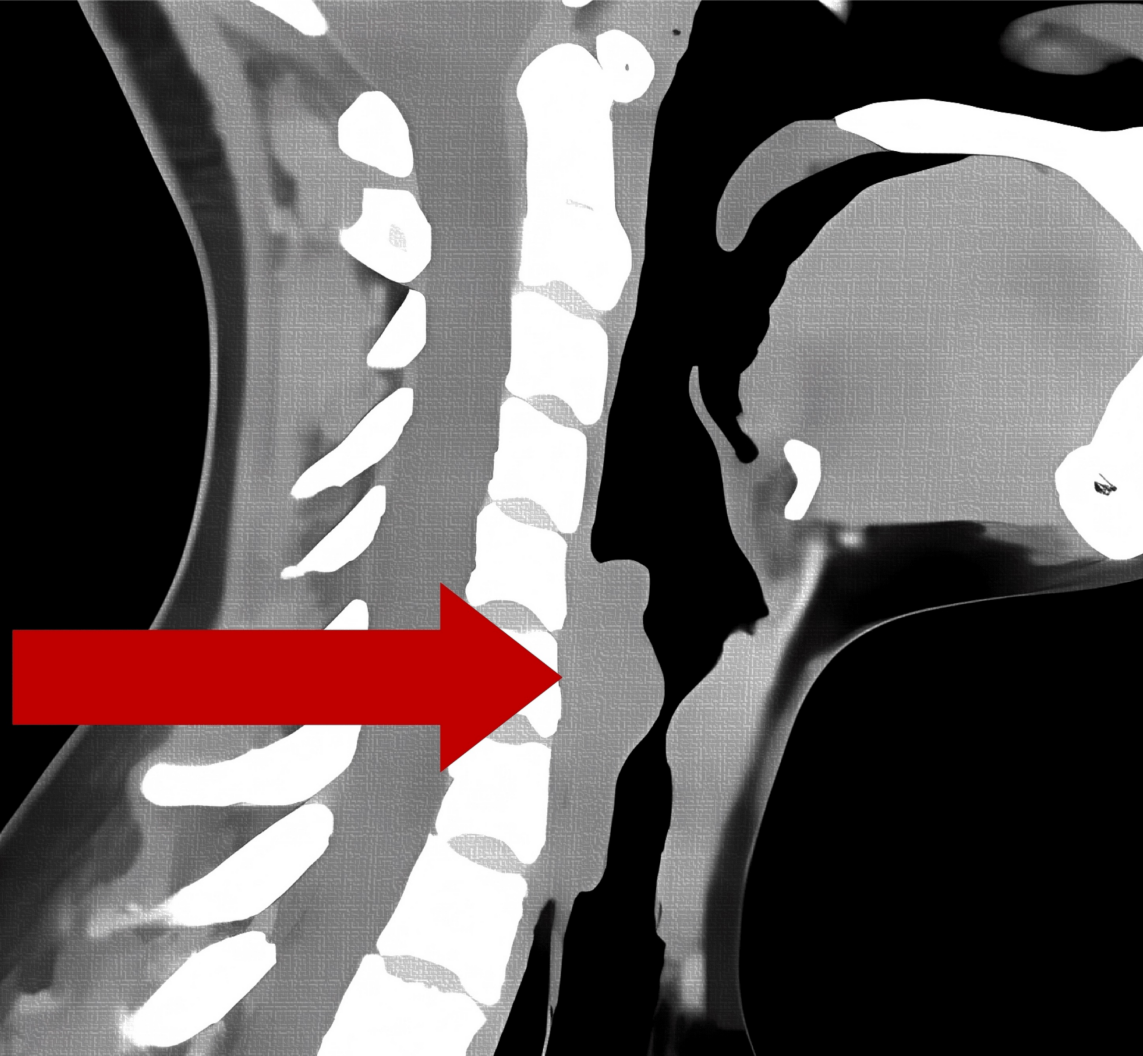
Computed tomography (CT) scan of the neck and chest showing severe tracheal stenosis with a luminal diameter of 5 mm. The imaging studies allowed the severity of the obstruction to be assessed and the appropriate surgical intervention to be planned.
